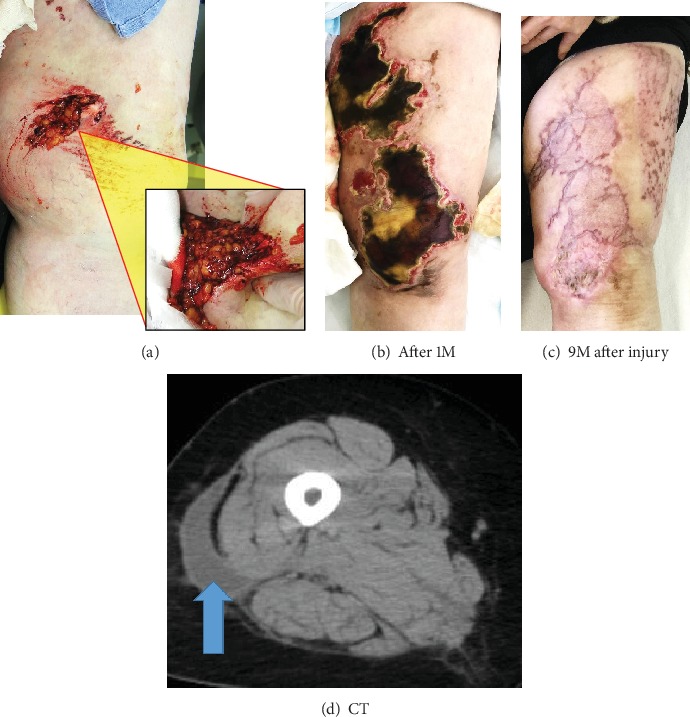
(a) Intraoperative photo. Fat masses came from a small size of wound. (b) Clinical photo. One month after the injury. Skin necrosis develops. (c) Clinical photo. Nine months after the injury. Skin grafts were performed, and the healing was complete. (d) CT scan performed three months after the injury. A small fluid collection between the subcutaneous soft tissue and the muscle fascia is indicated by an arrow.
